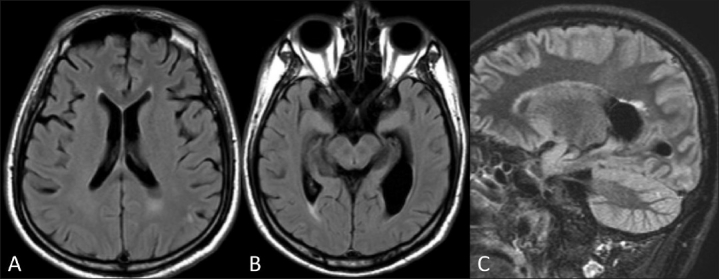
MRI of the brain in July. Axial FLAIR (A,B) and sagittal STIR-FLAIR (C) images show significant regression of the extent of the white matter involvement as well as the signal intensity
